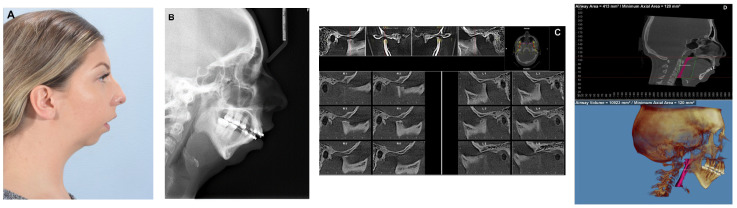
(A) 24-year-old female with bilateral condylar resorption, high occlusal and mandibular plane angles, retruded mandible, severe Class II appearance and anterior open bite. (B) Lateral cephalogram confirming clinical findings. (C) TMJ views from CBCT confirm severe condylar resorption. (D) Airway analysis shows severely decreased airway space (Airway volume = 10,923 mm^3^, Minimum Axial Area = 120 mm^2^).
